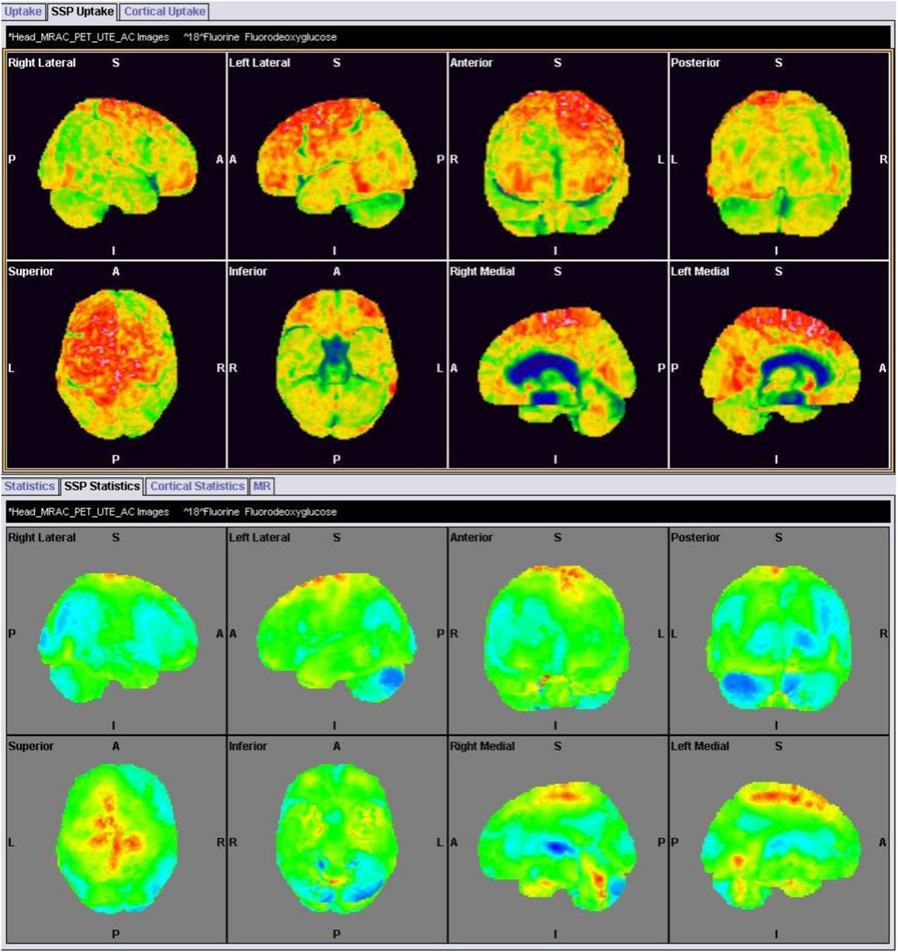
PET-MRI showing a right parieto-temporal and frontal hypo-metabolism; a bilateral hypo-metabolism of cingulate cortex is visible, as well.
